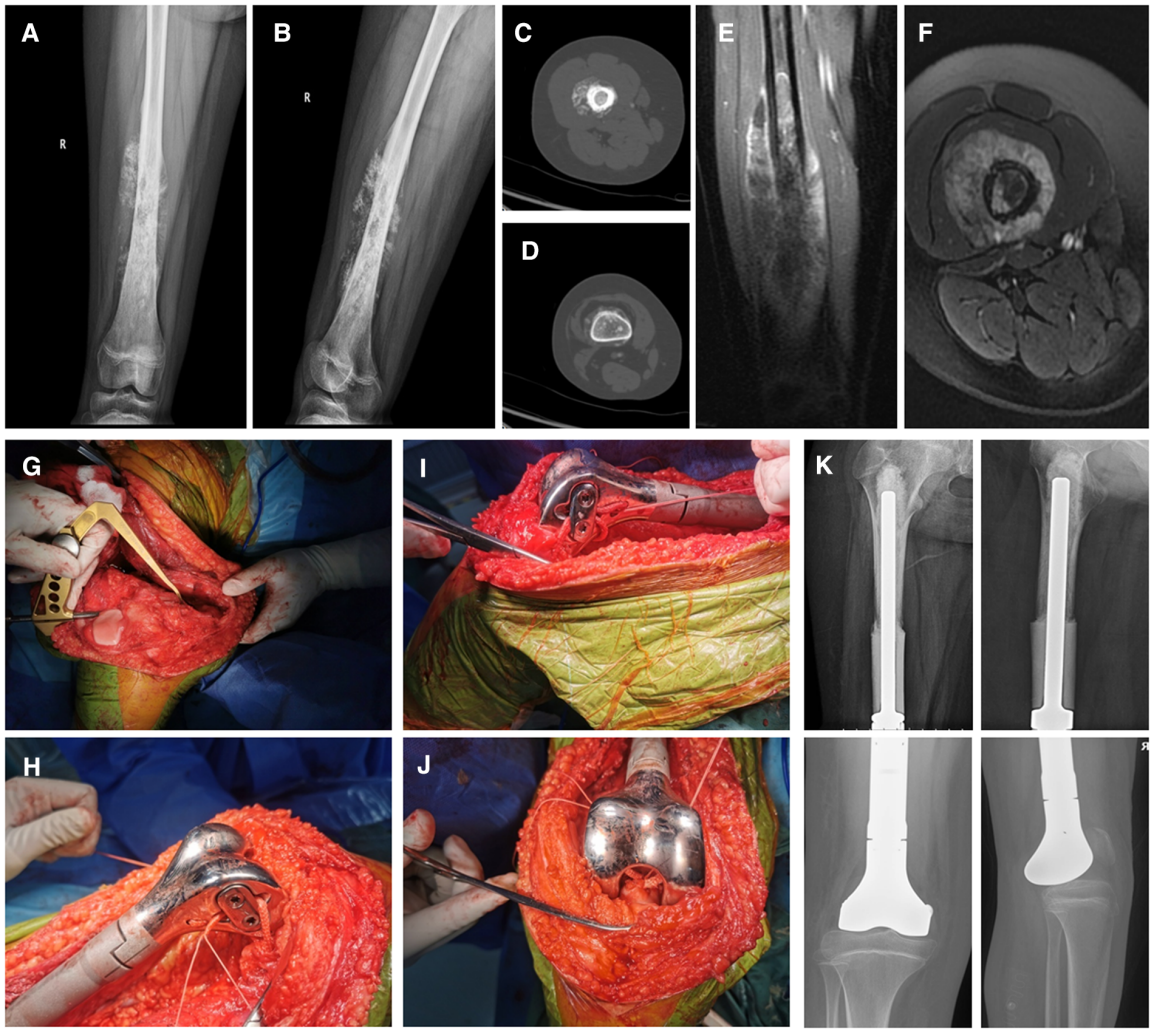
12 years old, female, osteosarcoma of the right distal femur, semi-joint prosthesis replacement combined ligament advanced reinforcement system (LARS) ligament reconstruction. (A,B) Preoperative radiographs of the femur revealed space occupation, bone destruction, and periosteal reaction of the right distal femur; (C,D) Preoperative CT revealed osteoblastic destruction of the right distal femur; (E,F) Preoperative MRI revealed an abnormal signal area in the right distal femur with a peripheral soft tissue mass and tumor invasion of the epiphyseal line; (G–J) The method of intraoperative LARS ligament reconstruction and its appearance after reconstruction; (K) Postoperative x-ray of femur indicated that the prosthesis was in good position.
